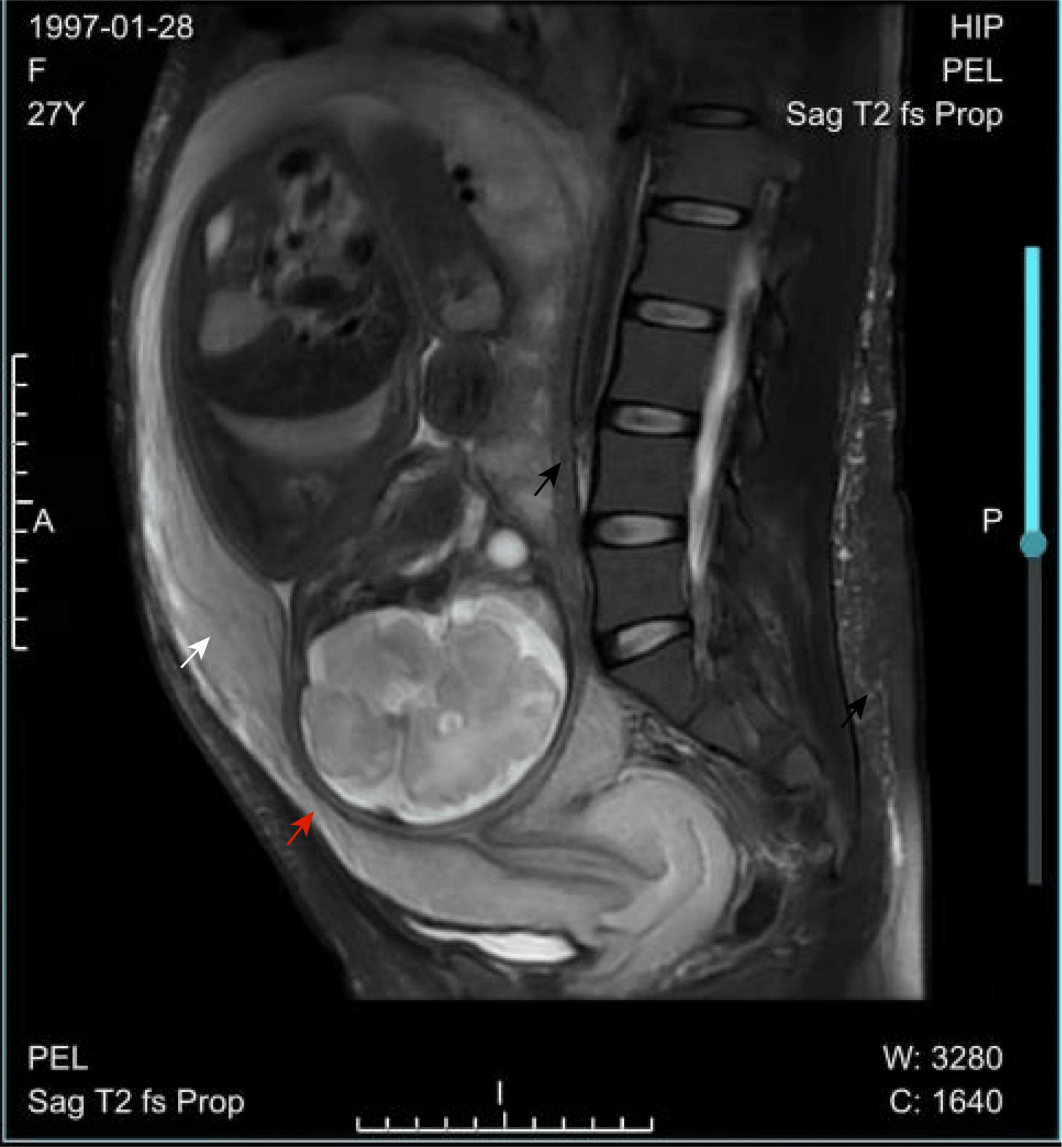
MRI of the pelvis indicated diffuse thickening of the uterine wall and the thinner lower uterine segment for the incision.
